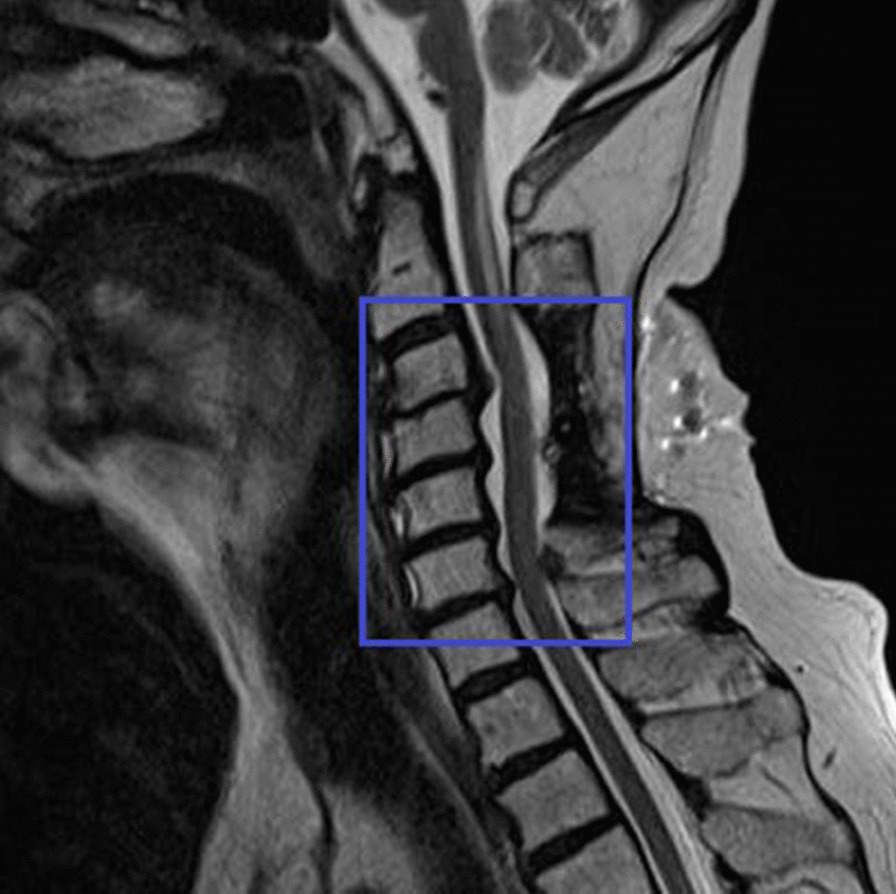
Magnetic resonance imaging of the cervical spine showing C3–C4 cervical spinal canal stenosis due to cervical spondylosis (inside the blue box)
